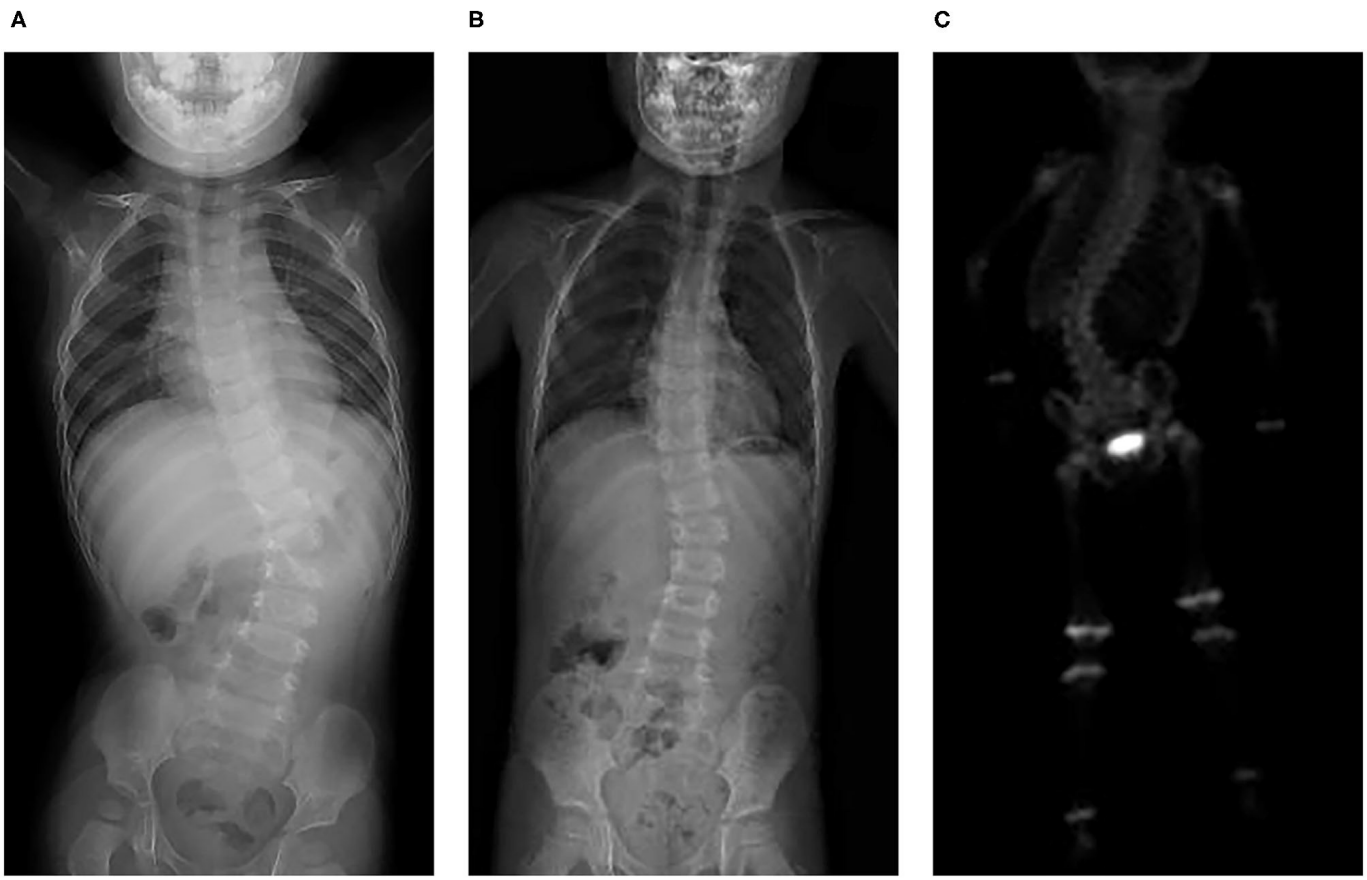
KHE in Patient 2: X-ray showed slight scoliosis (A), and the angle of the scoliosis was reduced after 3 years of treatment and follow-up (B). Three hours after 99 mTc-MDP 10 mCi was injected intravenously, a whole-body bone scan was performed. It also showed scoliosis and slightly increased radioactive distribution on the right edge of the T12-L2 vertebral body (C).
